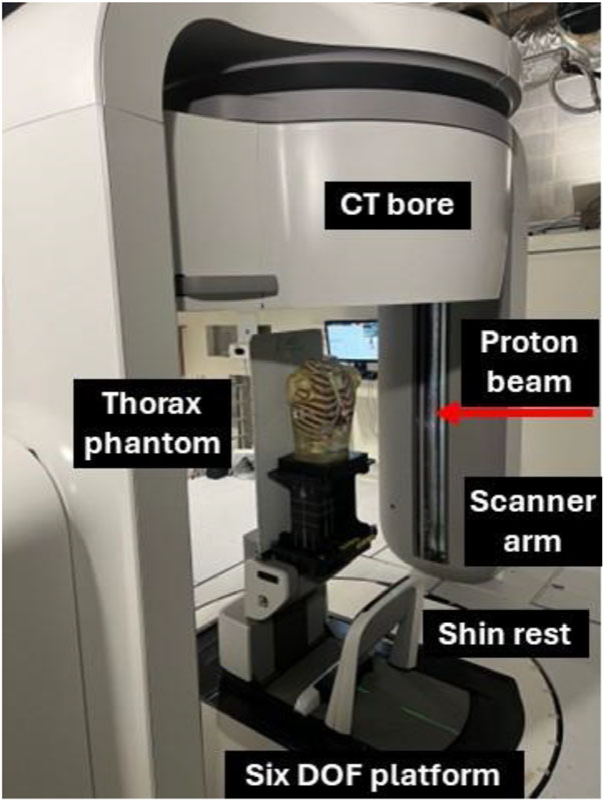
Upright CT system for radiation therapy.
